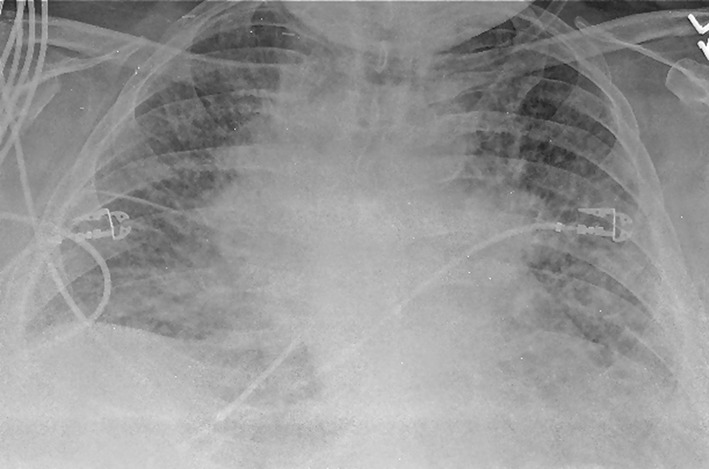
A repeat chest X-ray (day 7) revealed diffuse bilateral infiltrates or edema, predominantly interstitial in character with cardiomegaly (not significantly improved from admission, in spite of aggressive diuresis).
